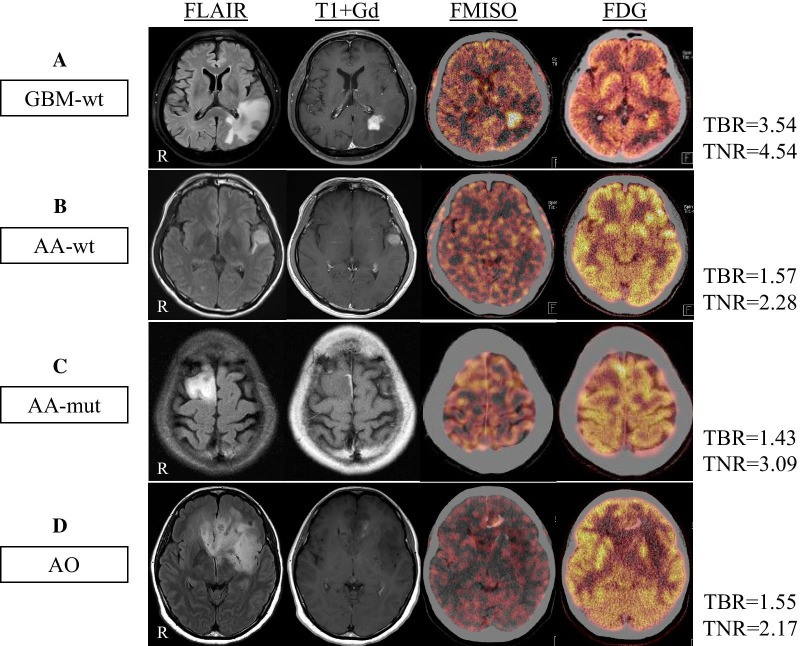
MRI (FLAIR and T1 + Gd) and PET images (FMISO and FDG) in representative glioma patients. a A 66-year-old male patient with GBM-wt showing high uptake of FMISO (TBR = 3.54) and FDG (TNR = 4.54). b A 36-year-old male with AA-wt showing slightly increased uptake of FMISO (TBR = 1.57) and FDG (TNR = 2.28). c A 31-year-old female with AA-mut showing slightly increased uptake of FMISO (TBR = 1.43) and high uptake of FDG (TNR = 3.09). d A 38-year-old female with AO showing slightly increased uptake of FMISO (TBR = 1.55) and FDG (TNR = 2.17)
